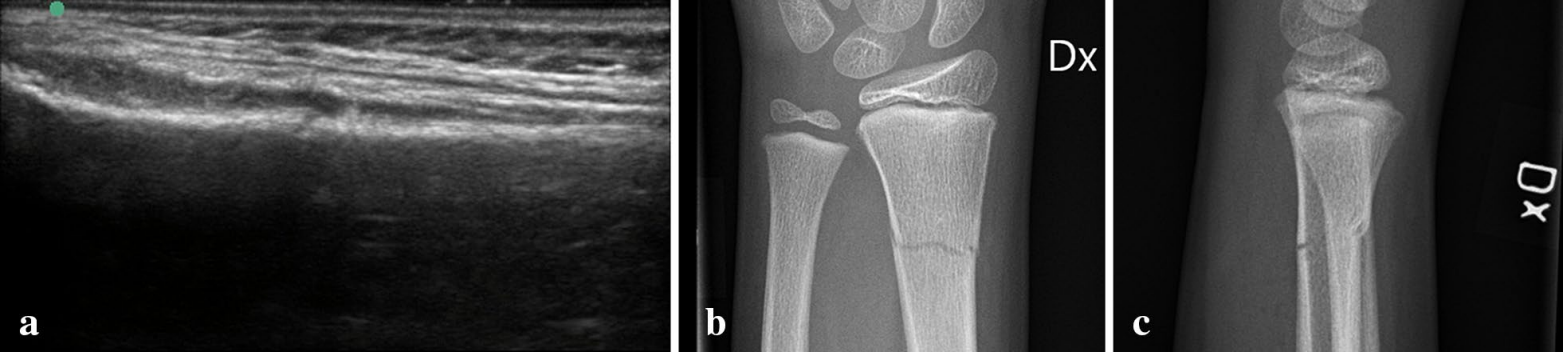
a Complete non-displaced fracture of the distal radius. US volar longitudinal view. Note the discontinuous *cortical line*. b, c (Same case as a) Complete non-displaced fracture of the distal radius on lateral and AP radiographs
